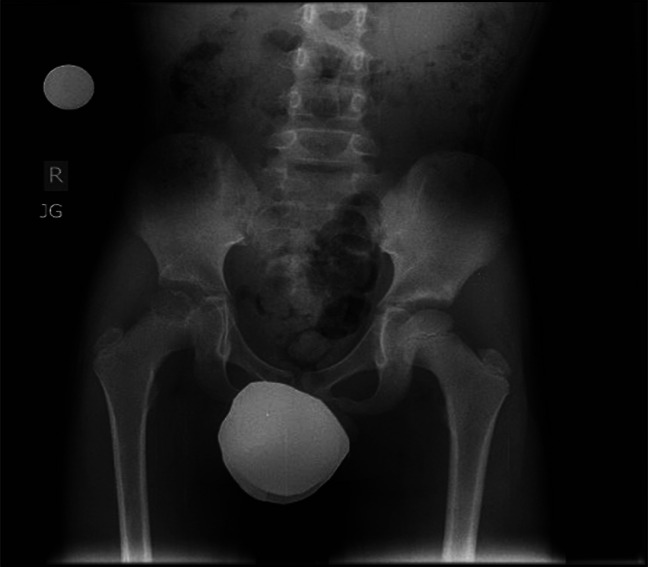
AP radiograph showing a well-defined radiolucent lesion in the epiphysis of the right proximal femur.
